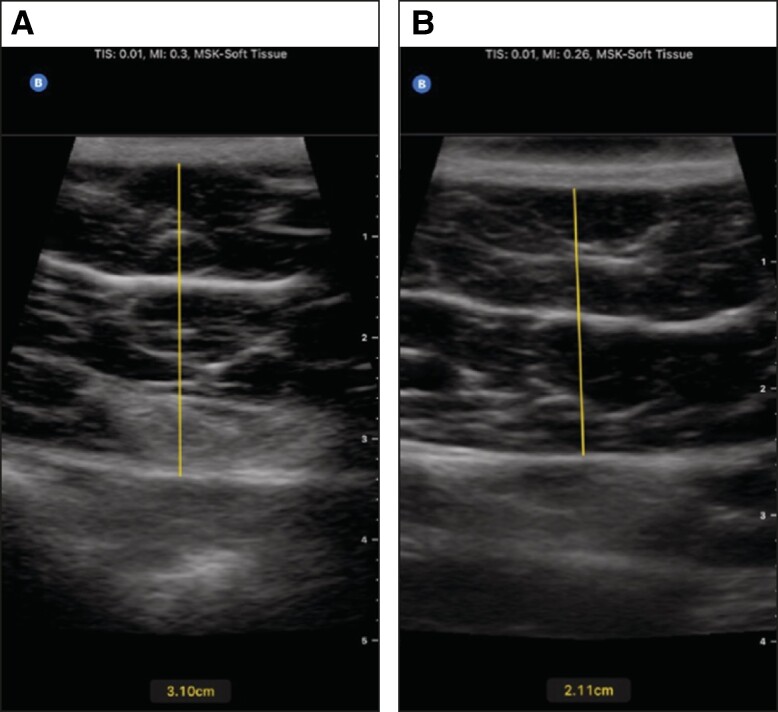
Ultrasound fat thickness measurements at baseline (A) and 3-month after (B) completion of the treatment series. The yellow line depicts the measured adipose thickness with the calculated measurement displayed in yellow font on each image.
